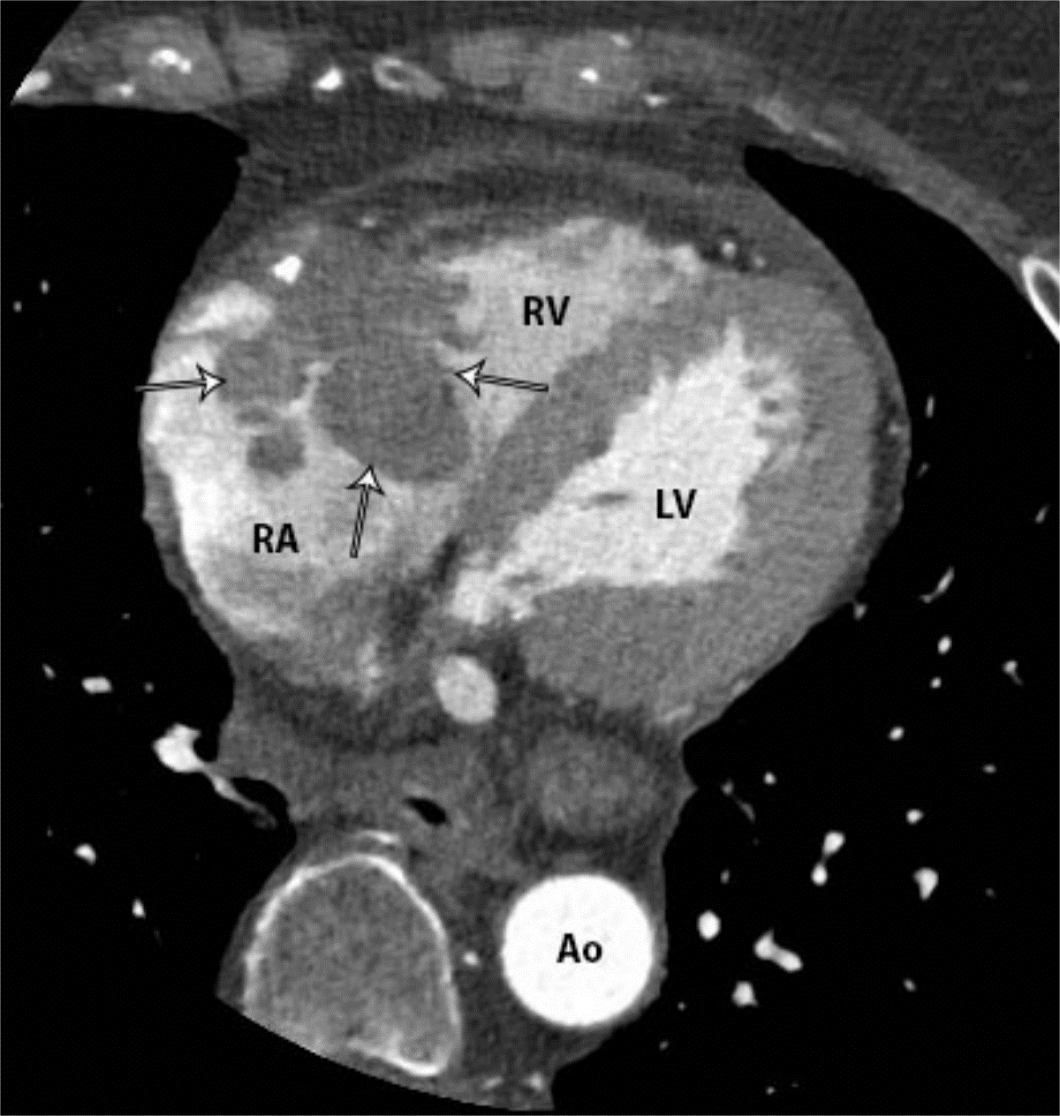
Axial coronary CT angiogram with contrast demonstrating a tricuspid mass (white arrows) extending into the right atrium and ventricle at the level of the AV canal, with narrowing of the right atrioventricular orifice and mild dilation of the right atrium. RA=right atrium; RV=right ventricle; LV=left ventricle; Ao=descending aorta.
